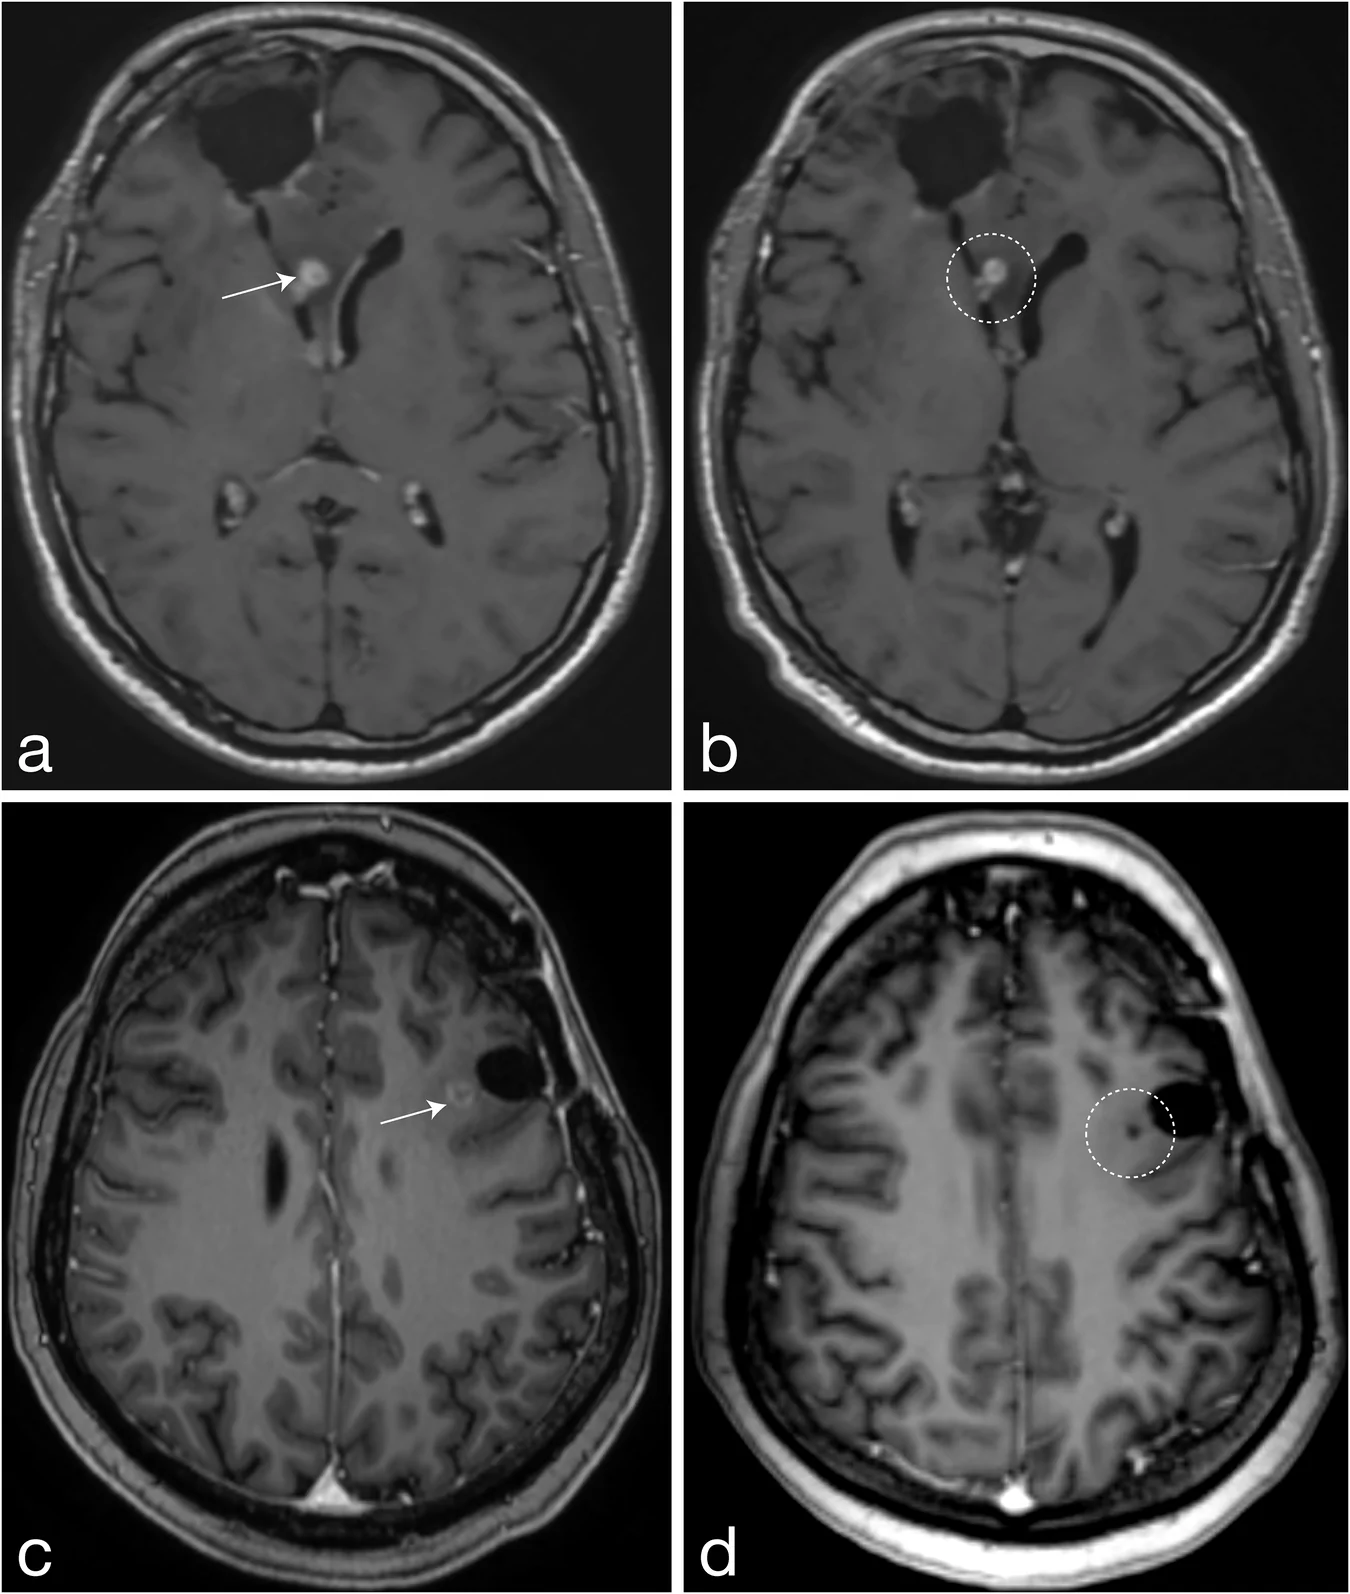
Delayed pseudoprogression in IDH-mutant glioma. Top row (Case 1): a patient with IDH-mutant astrocytoma treated with surgical debulking, radiotherapy and adjuvant PCV chemotherapy. Axial post-contrast T1-weighted images at (a) 6 months and (b) 9 months after completion of radiotherapy demonstrate a small punctate periventricular enhancing focus (arrow in(a; dashed circle in b) that remained essentially unchanged, possibly marginally smaller, on follow-up. Bottom row (Case 2): a patient with IDH-mutant glioma treated with biopsy, radiotherapy and adjuvant PCV chemotherapy. Axial post-contrast T1-weighted images at (c) 6 months and (d) > 2 years after completion of radiotherapy demonstrate a small pericavitary enhancing focus (arrow in c) with complete resolution on long-term follow-up; the dashed circle in d marks the prior lesion location
